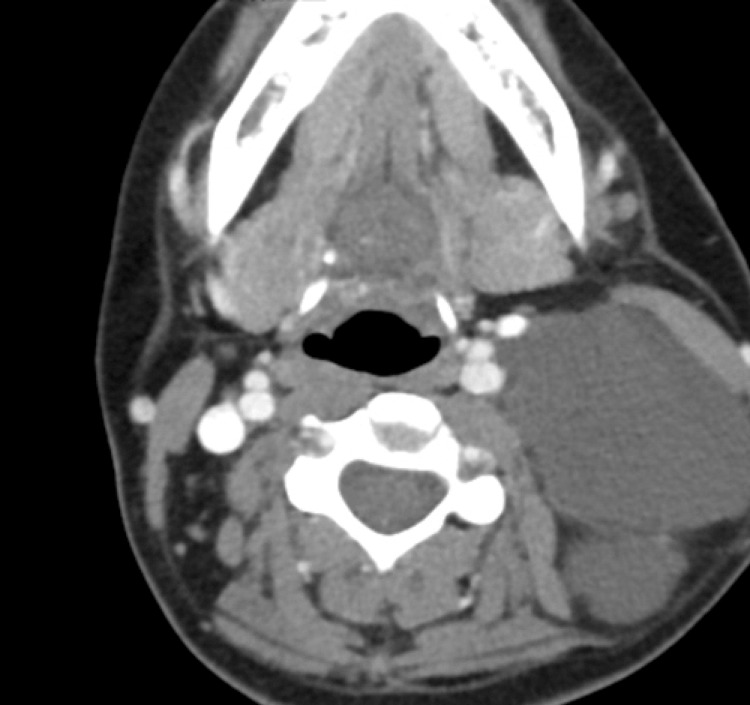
Axial CT scan of the neck
